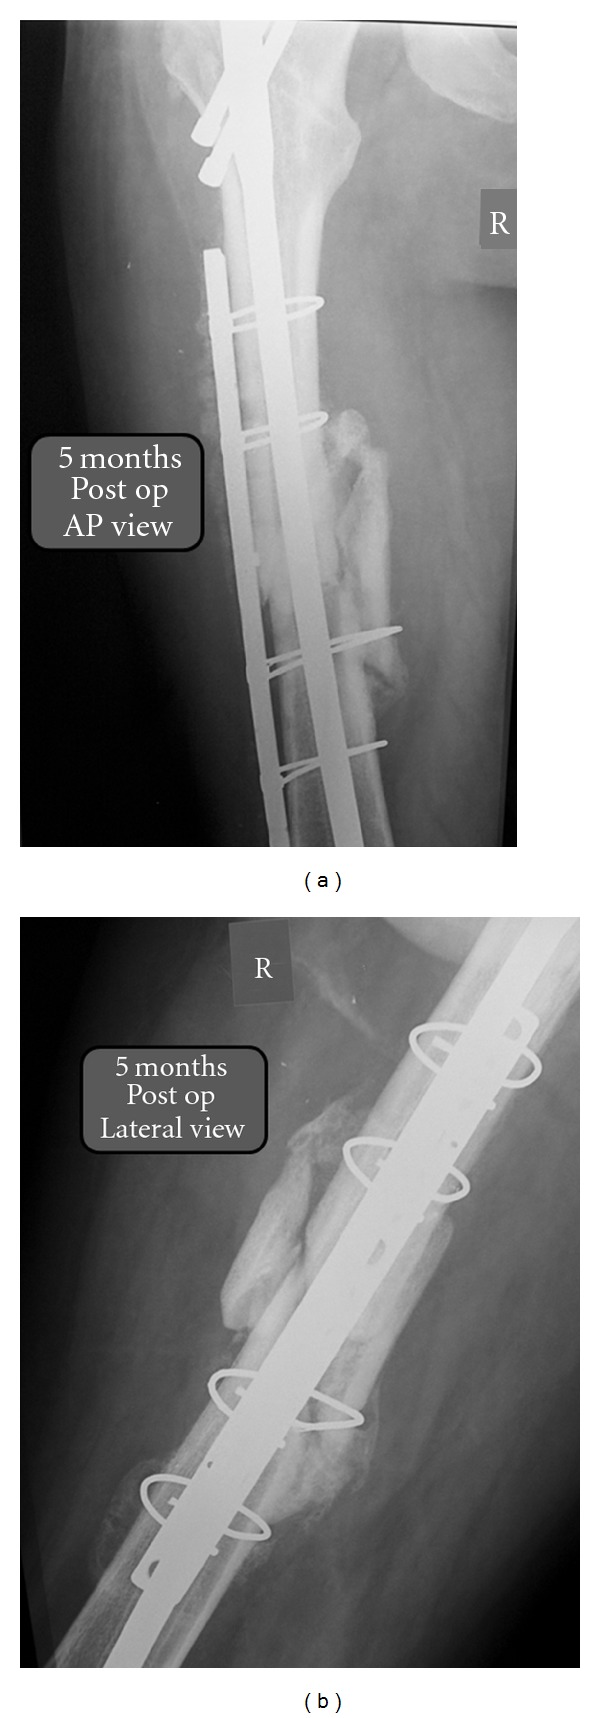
Radiographic imaging of femoral reconstruction five months postoperatively in (a) anteroposterior and (b) lateral view, showing progression of callus formation.
